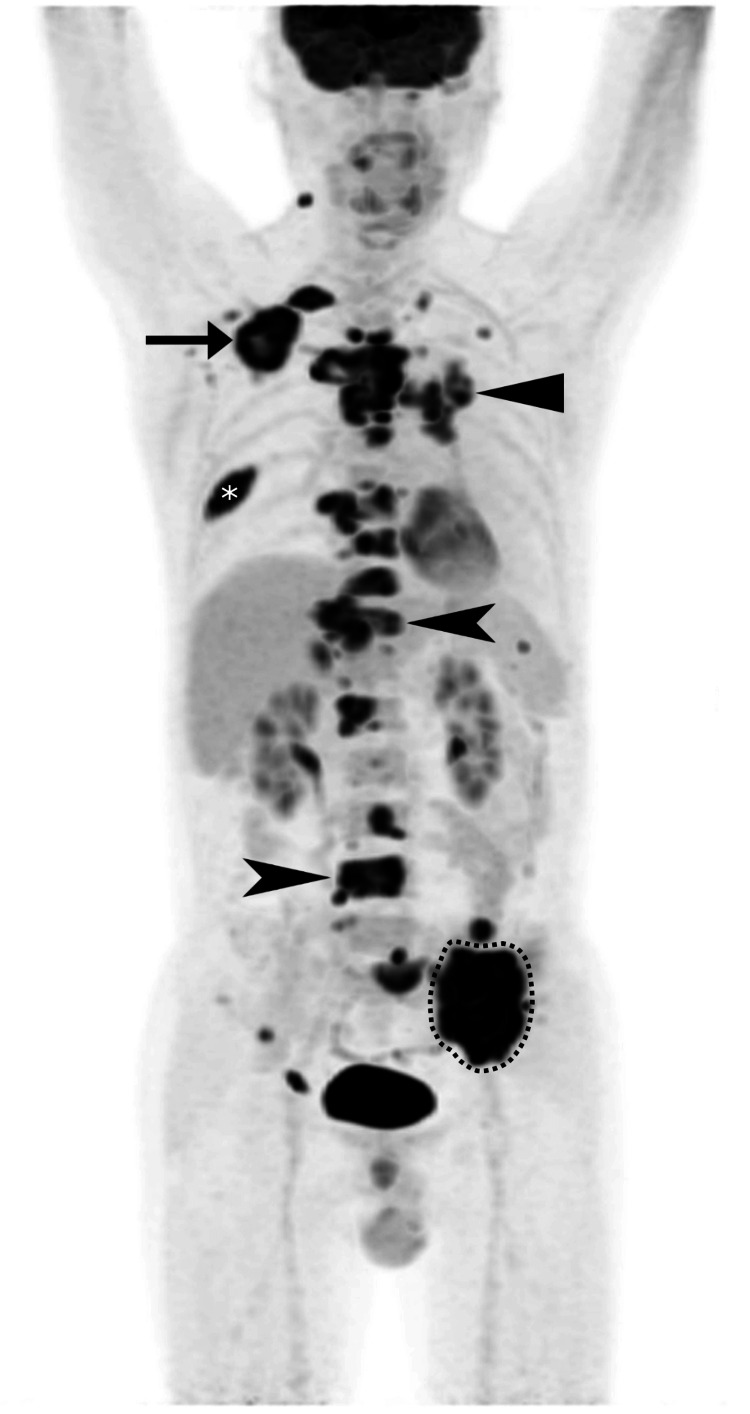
Coronal F18-fluorodeoxyglucose (FDG) whole body positron emission tomography/computed tomography (PET-CT) Increased FDG uptake is evident throughout several sites. Select representative lesions are highlighted with their corresponding maximum standardized uptake values (SUVmax) listed including the vertebrae (arrowheads) T11 (18.01) and L4 (20.42), right scapula (arrow; 20.69), right seventh rib (asterisk; 19.28), left ilium (dotted line; 25.17), and left medial upper lung lobe (triangle; 15.86).
